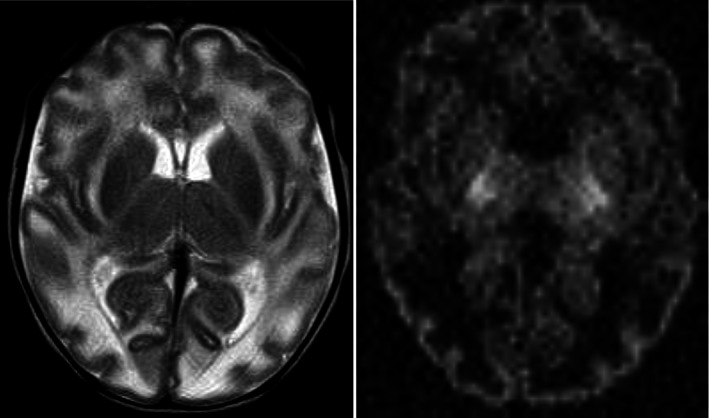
Clinical features and results of investigations. Magnetic resonance imaging of the brain findings at 14 days of age. The left panel axial T2 WI image shows high T2 signal in the cerebral white matter reflecting vasogenic oedema. No myelin deposition is seen in the posterior limbs of internal capsules bilaterally. The right axial DWI (diffusion‐weighted imaging) image demonstrates diffusion restriction in the thalamo‐capsular regions bilaterally in keeping with acute cytotoxic oedema.
